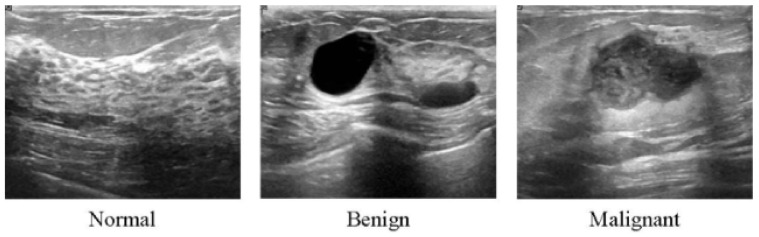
Ultrasound images from breast tissue for normal, benign, and malignant [80].
